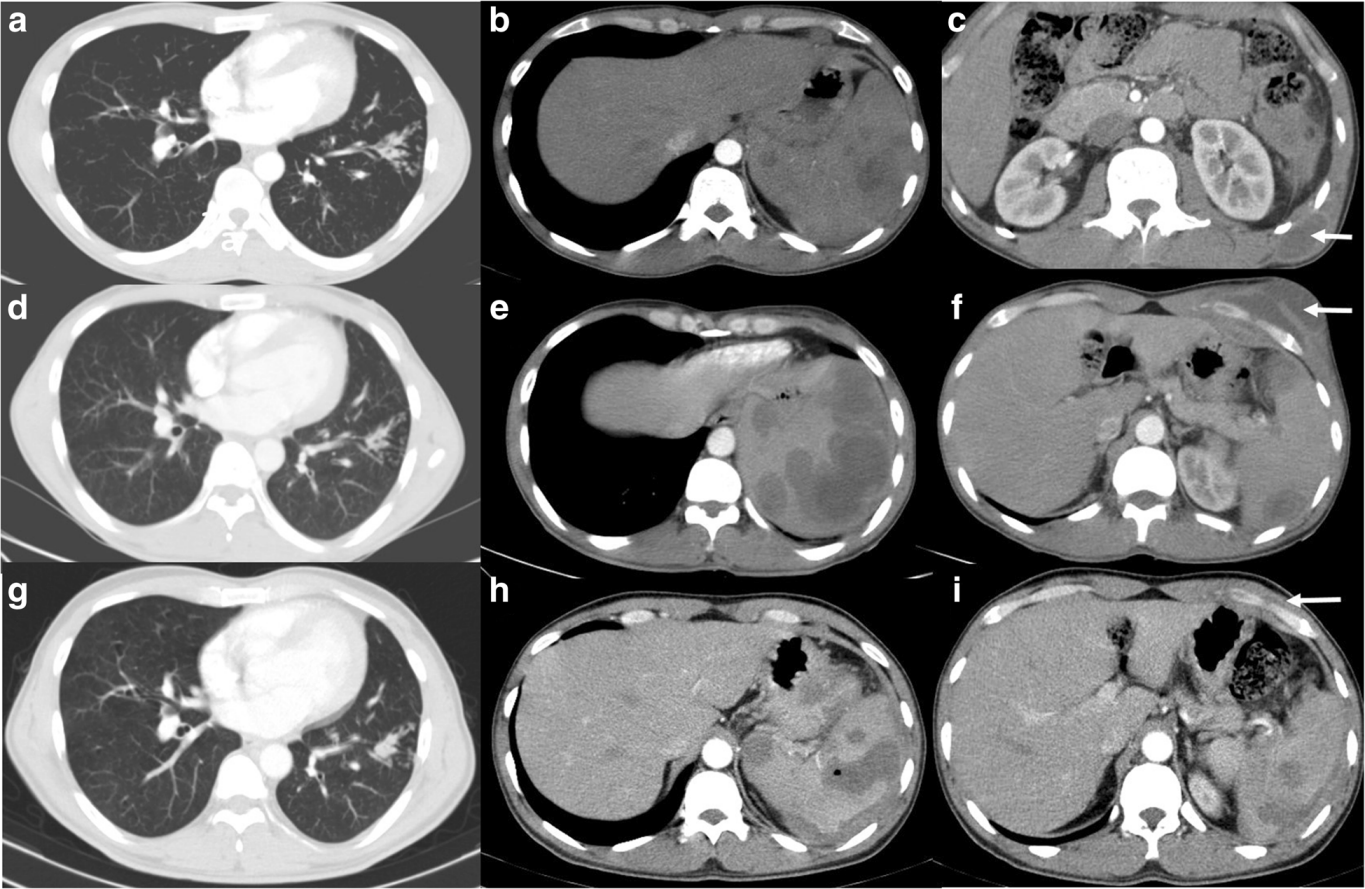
Chronic melioidosis with TB. Forty-one-year-old gentleman, newly diagnosed to have diabetes mellitus, presented with respiratory symptoms, (a) CT thorax showed the tree in bud opacities in the left lower lobe. Upper abdomen sections reveal (b) multiple hypodense foci in the spleen suggestive of small abscesses and (c) a small abscess in the left posterior lower chest wall (arrow). Cytology from the chest wall showed necrotising granulomatous inflammation, and antituberculous treatment was started. Two years later, he presented with new onset of left anterior chest wall swelling, for which CT thorax and abdomen were performed which showed (d) mild reduction in the ‘tree in bud’ opacities, but (e) increase in the size and number of splenic abscesses and (f) interval development of the left anterior lower chest wall abscess (arrow). Pus culture from the chest wall grew *Burkholderia pseudomallei*. Follow-up CT after 6 months showed, (g) stable tree in bud opacities in the lung, (h) near complete resolution of the chest wall abscess (arrow) but with the presence of perisplenic fluid with persisting splenic lesions (h and i), suggestive of contained rupture
